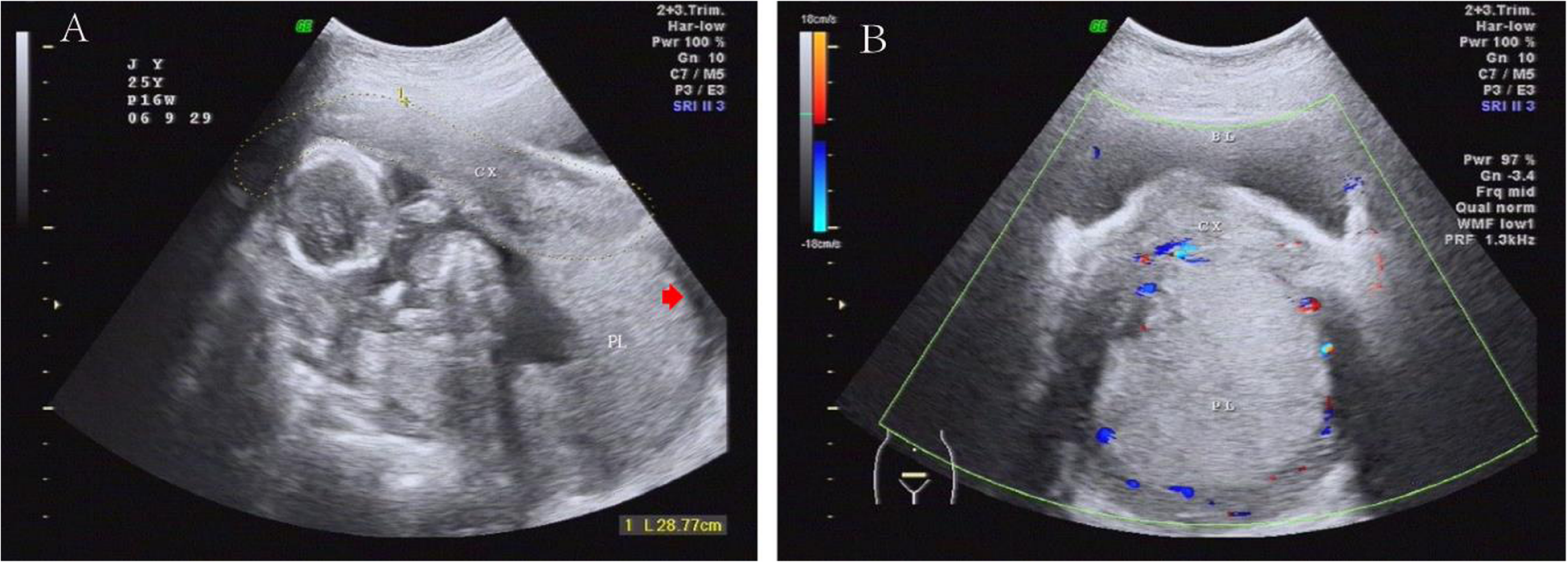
Ultrasonography of the retroverted gravid incarcerated uterus in the present case. (a) A longitudinal section shows that the uterus is fixed in retroversion with the cervix anteriorly transfixed behind the pubic symphysis above the uterine fundus (red arrow indicating the uterine fundus). Placenta located at fundus of uterus. (b) A transverse image of placenta that is located below the cervix. The two positional images better defines the position of uterine retroversion. BL: bladder; CX: cervix; PL: placenta
